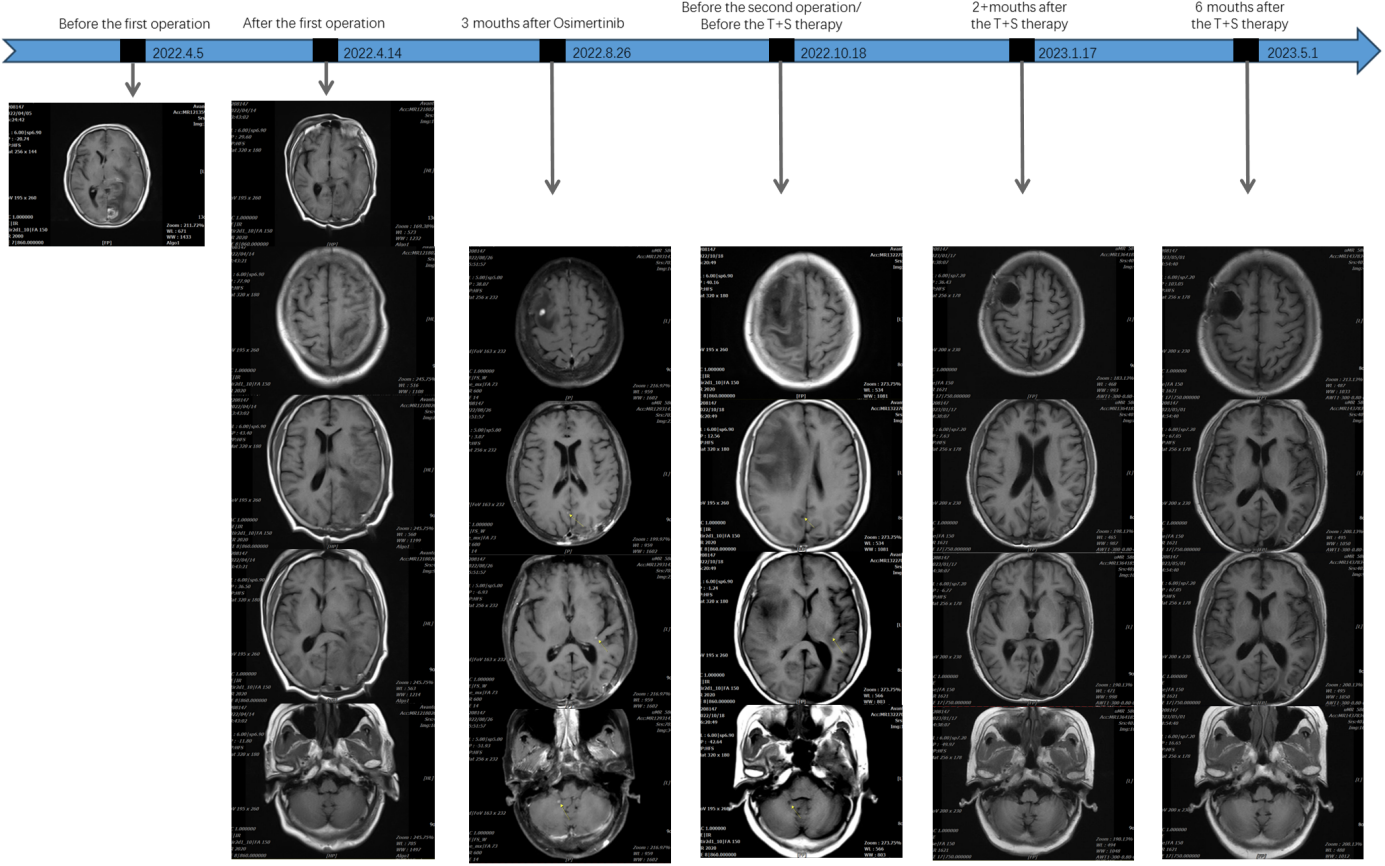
Brain MRI of the patient at various time points of the patient’s treatment.
